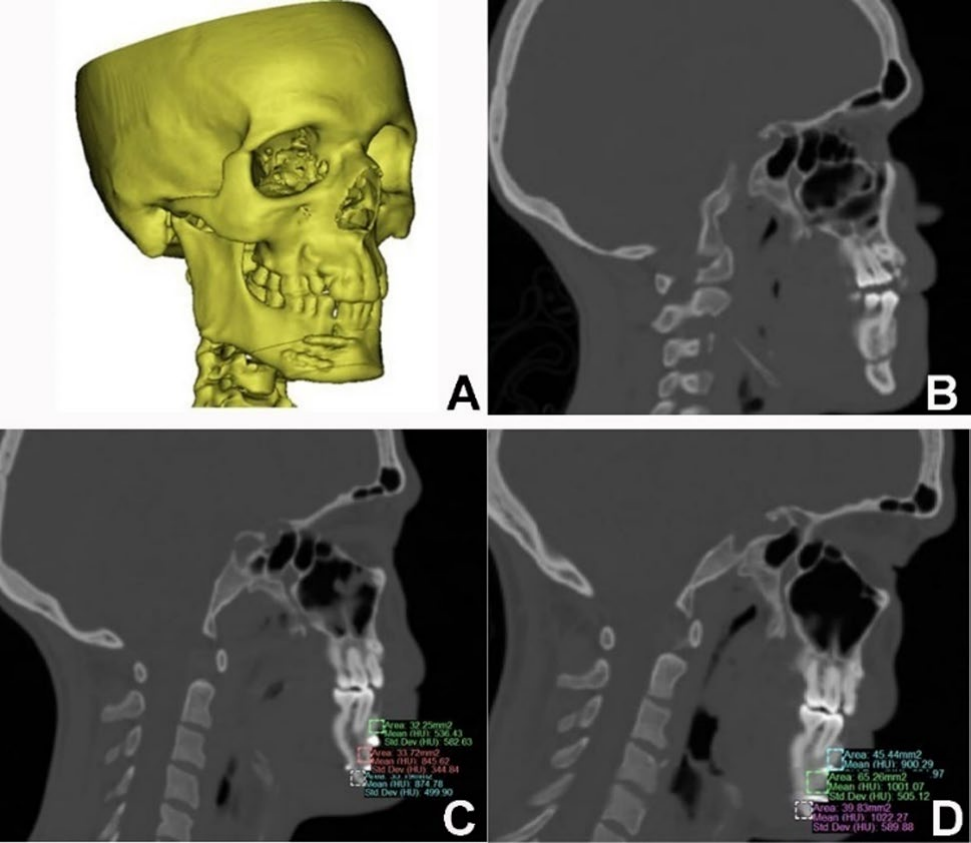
(Control case): A) 3D reconstruction of mandible treated with conventional 2 miniplate, B) preoperative CT, C) measuring bone density at immediate postoperative CT, D) measuring bone density after 6 months follow up CT
